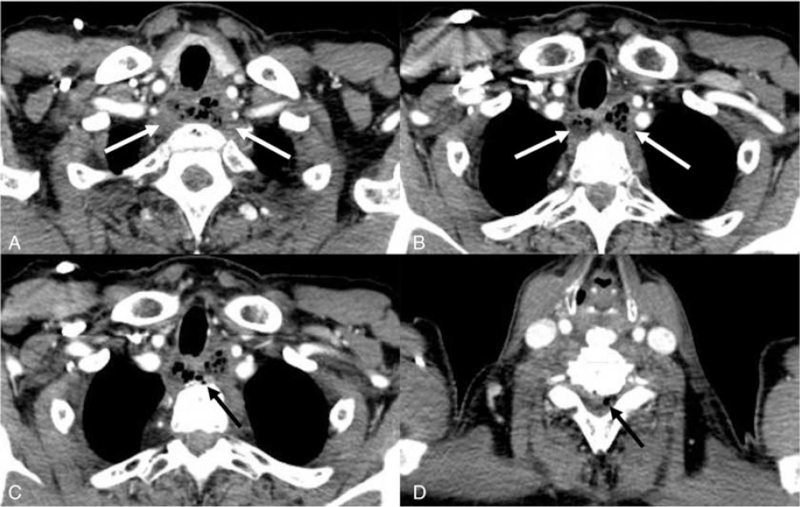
(A and B) Contrast-enhanced chest computed tomography (CT) revealed a diffuse, poorly enhanced esophageal lesion with necrosis and a mottled appearance (arrow), although there was no obvious esophageal defect or perforation. (C) On reviewing the chest CT scans after neurological symptoms appeared, small amount of free air (arrow) was apparent in the prevertebral space (D); a small amount of free air (arrow) was noted in the spinal canal.
